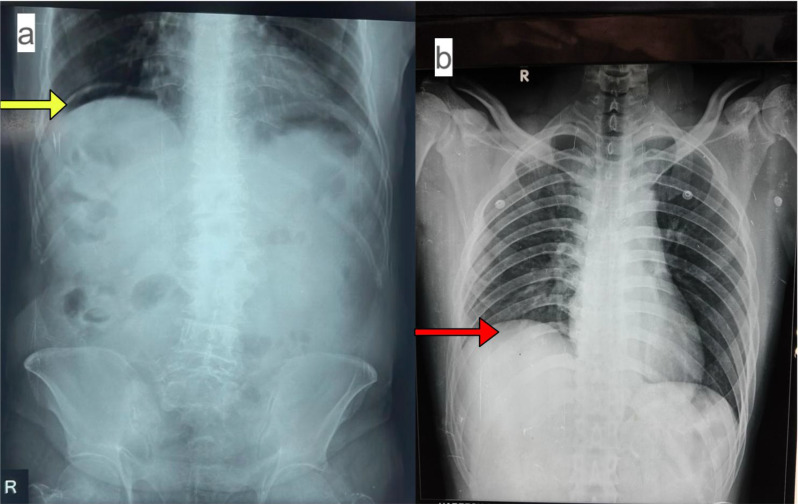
Upright abdominal radiographs. (a) Patient 1: Presence of subdiaphragmatic free air (yellow arrow). (b) Patient 2: Absence of visible subdiaphragmatic free air (red arrow)
